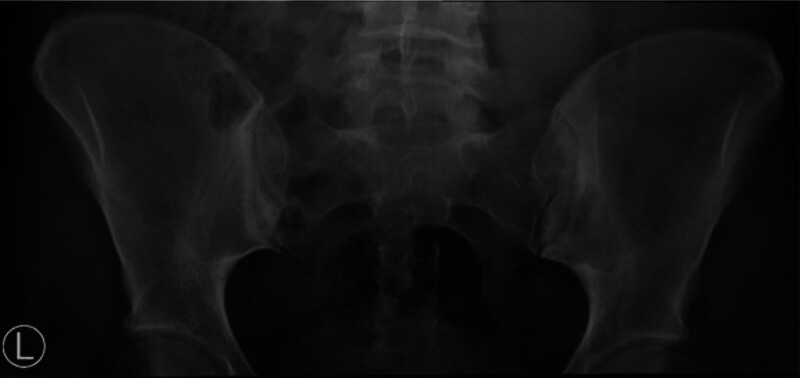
Anteroposterior radiograph of the pelvis demonstrating bilateral sacroiliac joint sclerosis with irregular joint margins and joint space narrowing, findings consistent with chronic structural sacroiliitis grade 2. No complete ankylosis was observed.
